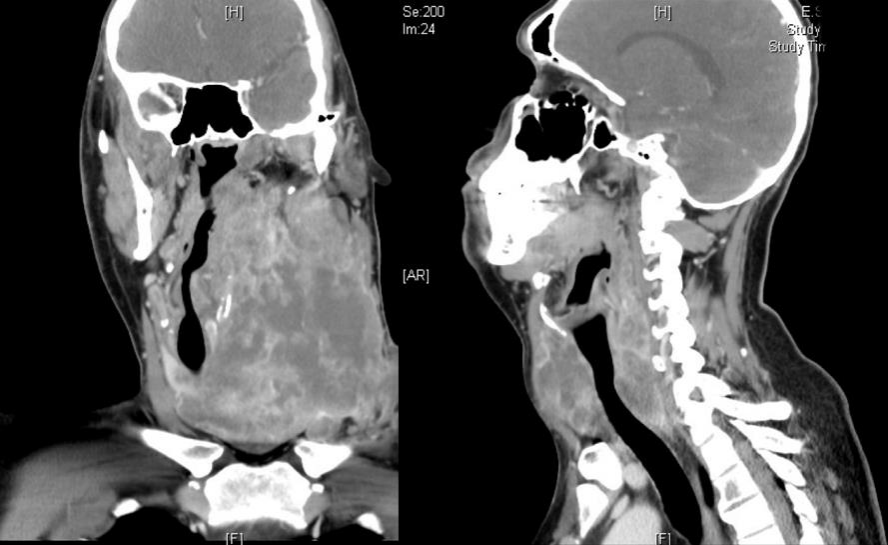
A CT scan showing a large left cervical mass displacing the trachea and vascular structures of the neck
